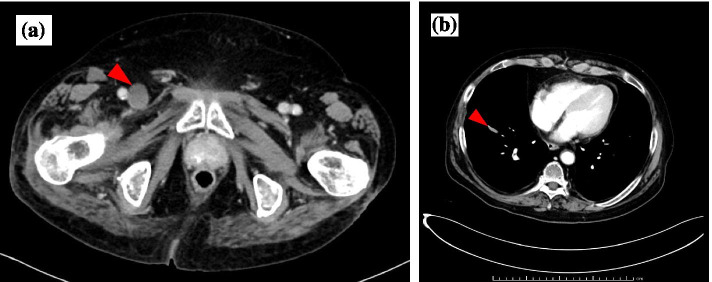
Computed tomographic images. Computed tomography revealed a thrombosis in the common iliac vein, causing dilation and occlusion of the vein, and b micro-thromboembolism in the pulmonary artery within the right lower lobe
